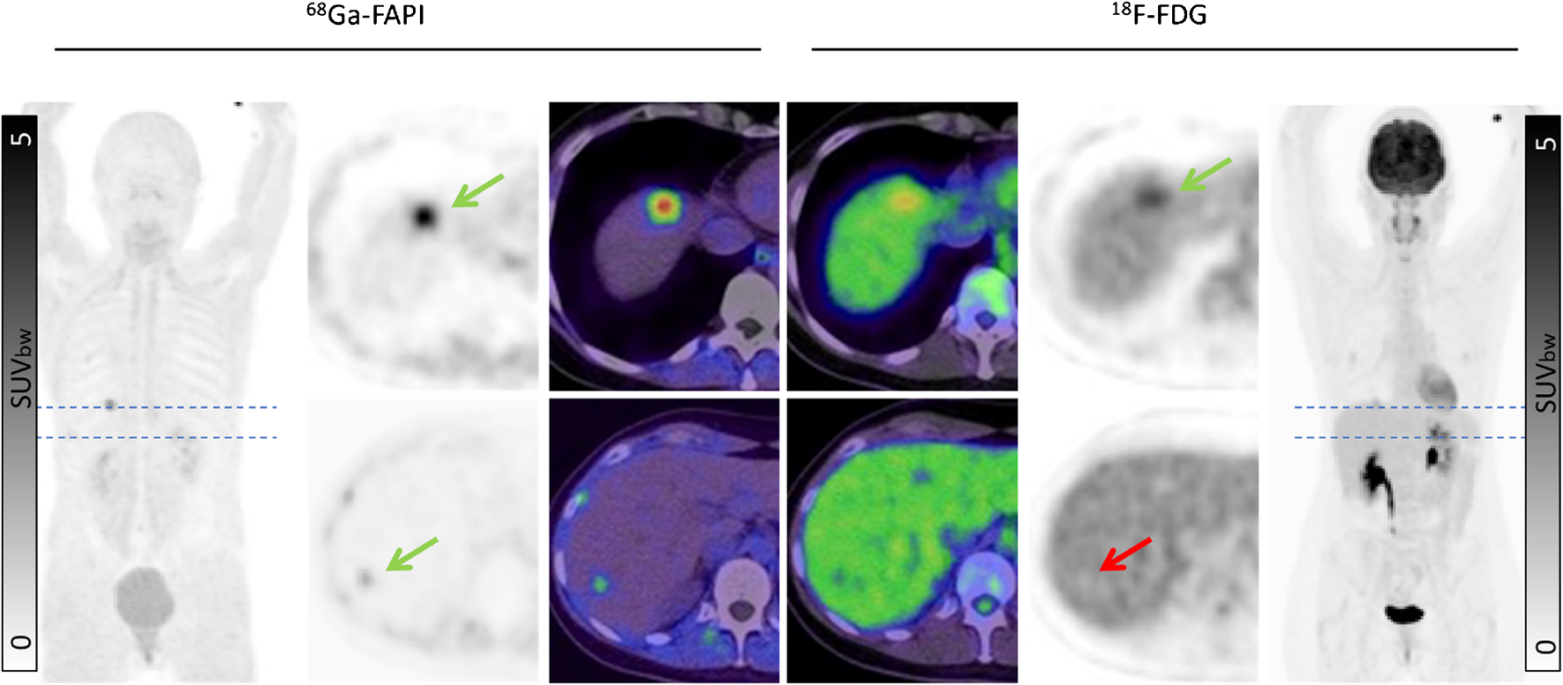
A 40-year-old female patient with ovarian cancer underwent restaging due to a suspicious subdiaphragmatic hepatic lesion. Tracer uptake in the normal liver parenchyma was markedly different on the two tracers: ^68^Ga-FAPI SUVmax 0.79 vs. ^18^F-FDG SUVmax 2.69. In the liver segment III and VII, a strong ^68^Ga-FAP uptake was found (green arrow) compared to ^18^F-FDG. The liver metastatic lesion in segment VII was not detected by ^18^F-FDG (red arrow)
